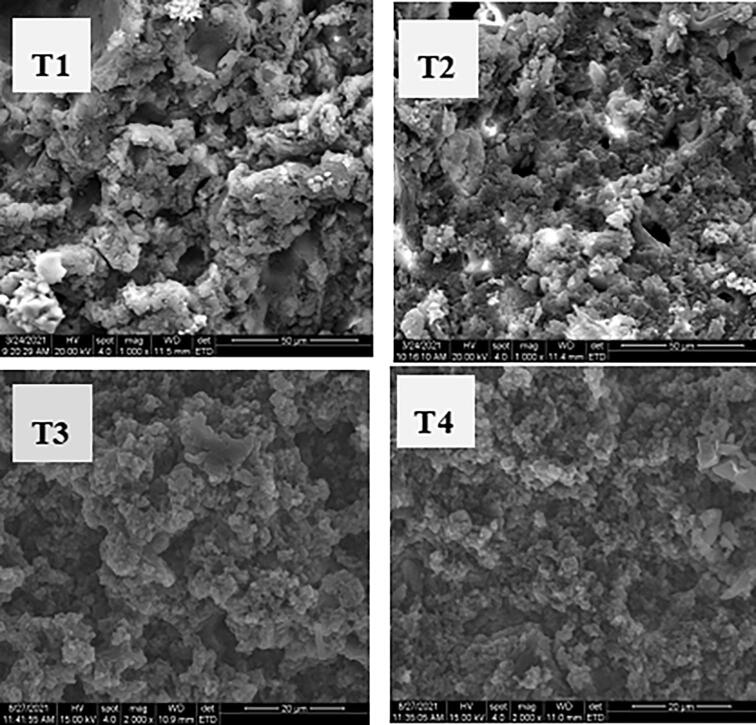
Effects of ultrasound-assisted sodium bicarbonate treatment on the microstructure of cooked pork batters. T1, sodium bicarbonate 0 g, ultrasound time 0 min; T2, sodium bicarbonate 2 g, ultrasound time 0 min; T3, sodium bicarbonate 2 g, ultrasound time 30 min; T4, sodium bicarbonate 2 g, ultrasound time 60 min.
